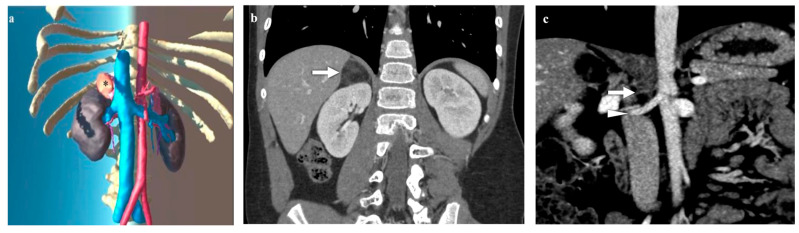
Virtual reality (VR) (a) and coronal computed tomography (CT) (b,c) images of a 7-year-old boy with a right adrenal myelolipoma (* in a; → in b) measuring 38 × 25 × 25 mm (AP × LL × CC), with heterogeneous density and fatty components. Images depict vascular details: bilateral accessory renal arteries, which on the right course antero-superiorly to the main renal artery heading towards the upper pole, while on the left run postero-superiorly parallel to the main renal artery.
